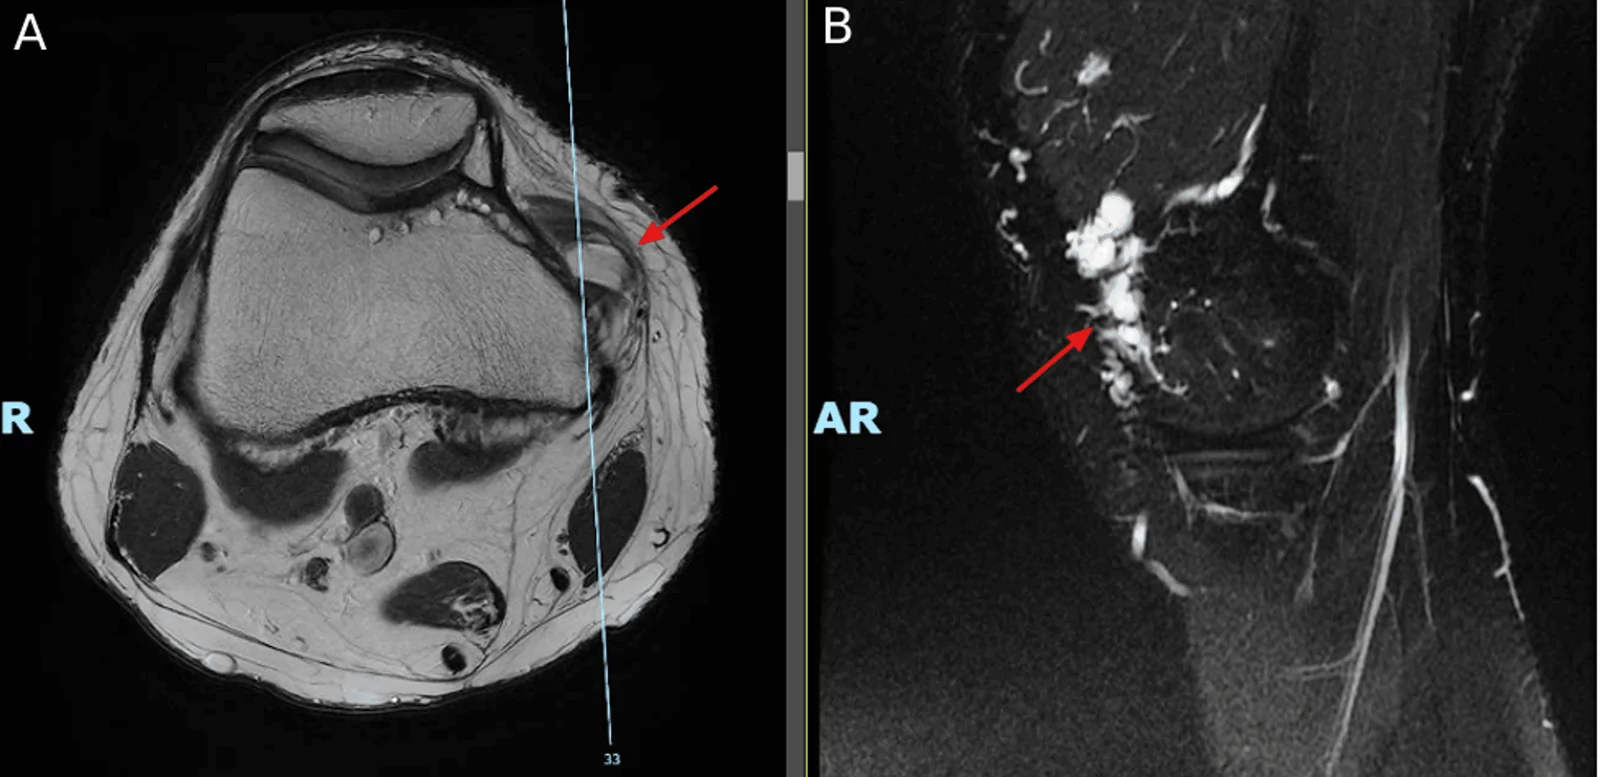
MRI of the right knee showing clusters of dilated, tortuous vessels, some focally dilated, extending from the vastus medialis muscle into the pre-femoral and Hoffa's fat pad, as well as extending into the femoral trochlea, suggestive of a slow-flow vascular malformation such as a venous malformation. (A) Transverse plane. (B) Sagittal plane.
